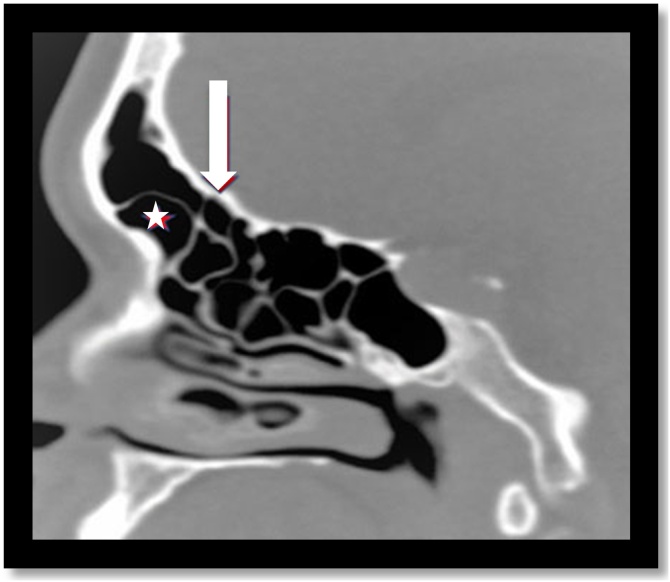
Supra-Agger frontal cell. Sagittal computed tomography section demonstrates an example of supra-Agger frontal cell (star) and supra bulla frontal cell (white arrow).
